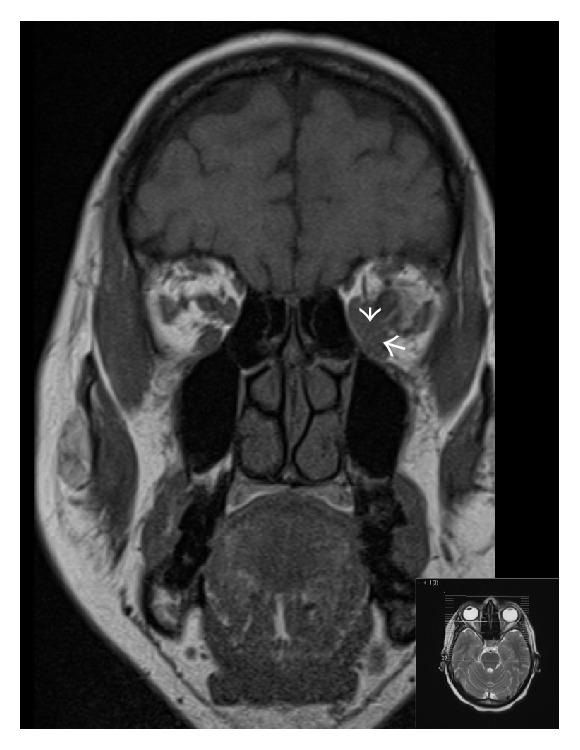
T1 weighted MRI orbits show soft tissue replacing fat in the medial left orbit is isointense with the adjacent recti and it is suggestive of a soft tissue deposit (white arrows).
